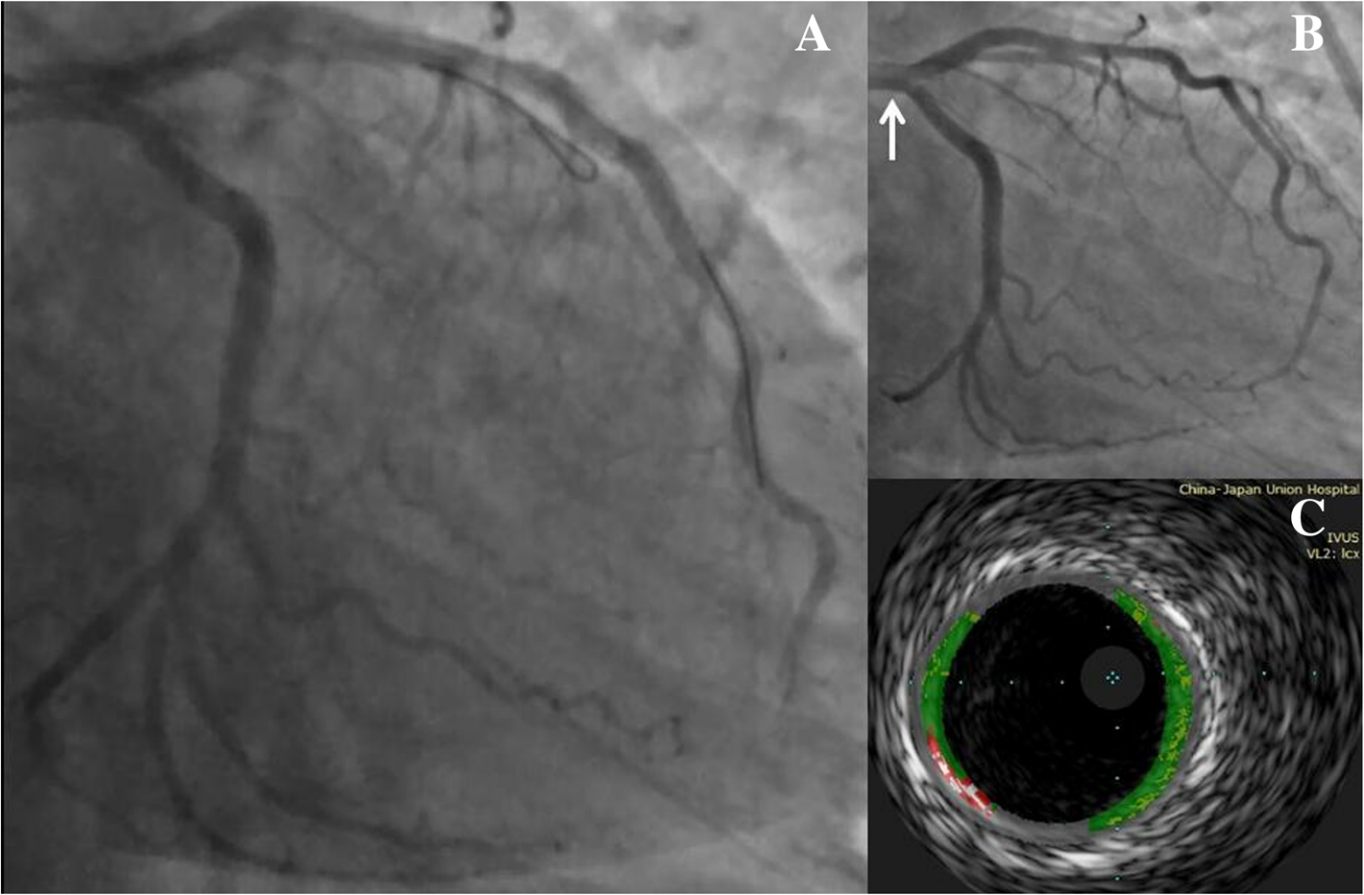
Final result and follow-up. a The final angiogram showed that only the terminal left anterior descending artery (LAD) was occluded by the thrombus. b. No angiographic signs of the residual thrombus in LAD were revealed in follow-up angiography. c Intravascular ultrasound (IVUS) showed non-significant residual stenosis of the left main coronary artery
